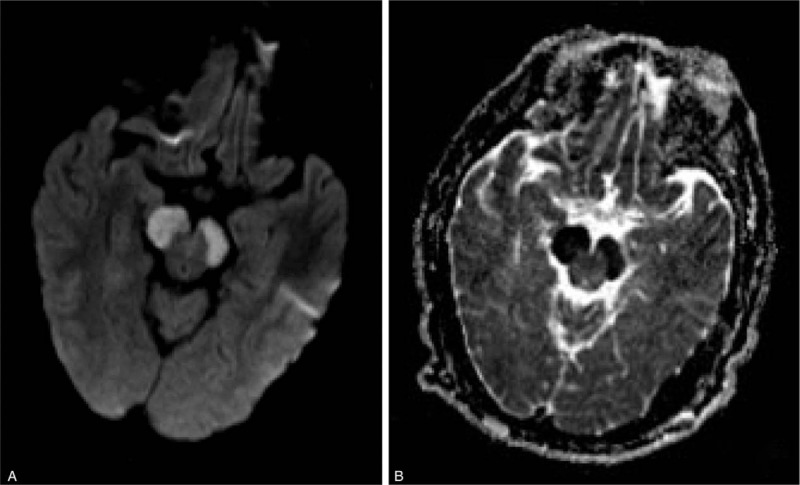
Re-examination of DWI (A) and ADC (B) on the eighth day of hospitalization.
